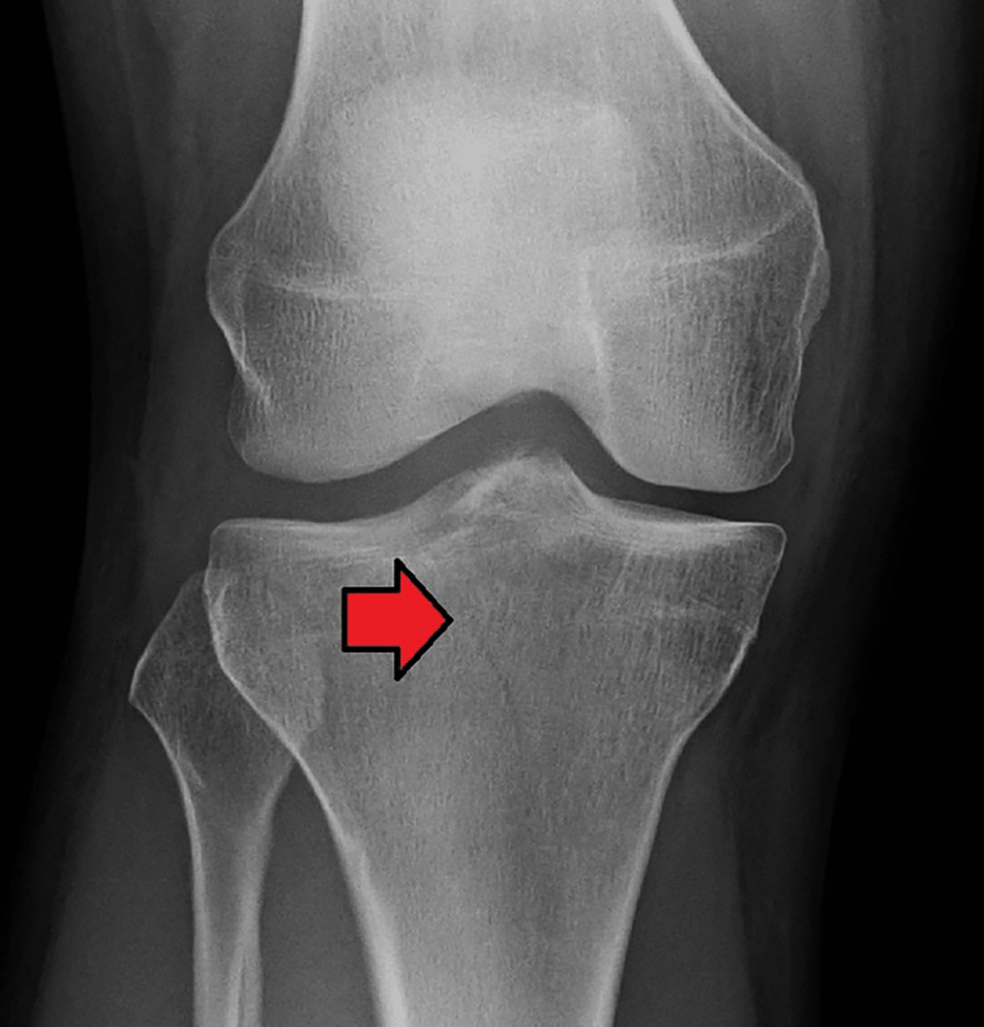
Anteroposterior view radiograph in plane of the plateau showing a subtle, Schatzker Type I tibial plateau fracture (arrow).
